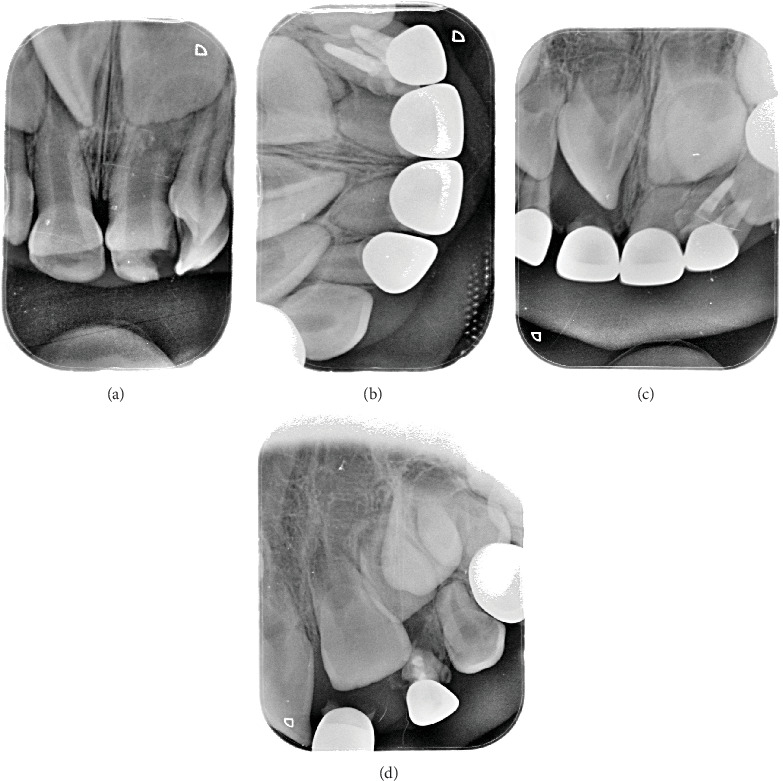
Triple fusion Type Ia. (a) Preoperative x-ray. (b) Postoperative x-ray. (c) 2.5 years follow-up. (d) 3.5 years follow-up.
